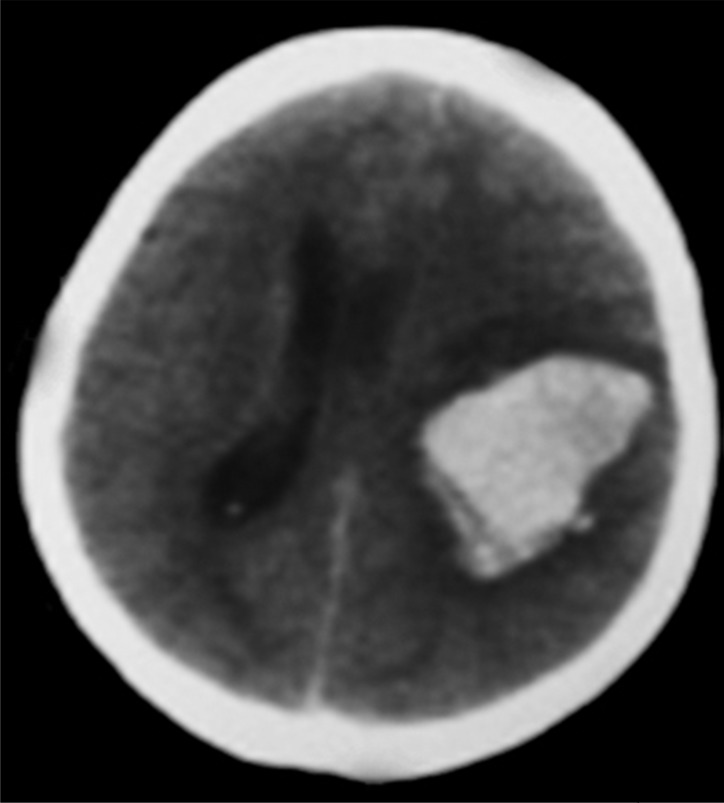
Brain computed tomography scan in the 4^th^ month of pregnancy showed left parietal lobe intra-cerebral hemorrhage
